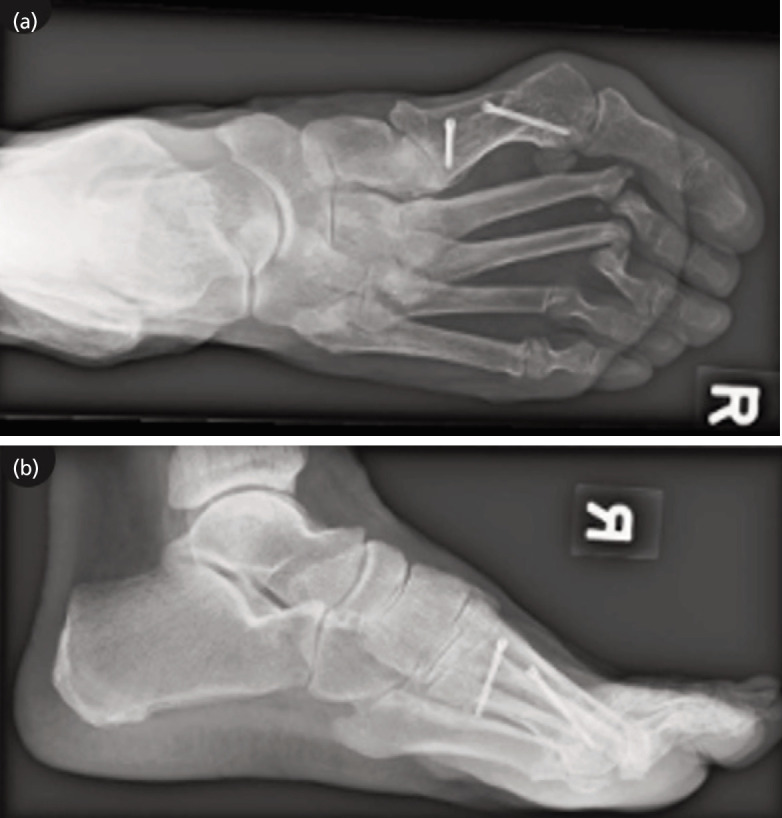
(a) AP and (b) lateral radiographs of the right foot showing a HV deformity with destruction of the MTPJs and degenerative changes of the first MTPJ and midfoot.
